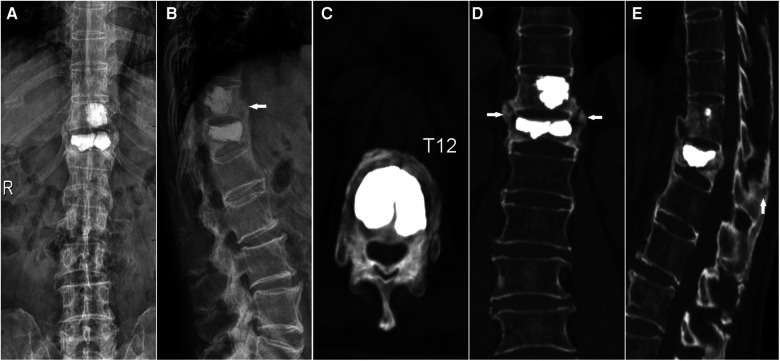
Twelve months later, bone union was observed (A,B). Traverse CT (C) showed union within the vertebra. Coronal CT showed the formation of a bone bridge (D). Sagittal CT showed intraspinous fusion (E). These ossification phenomena suggested that KP contributed to spinal stability.
